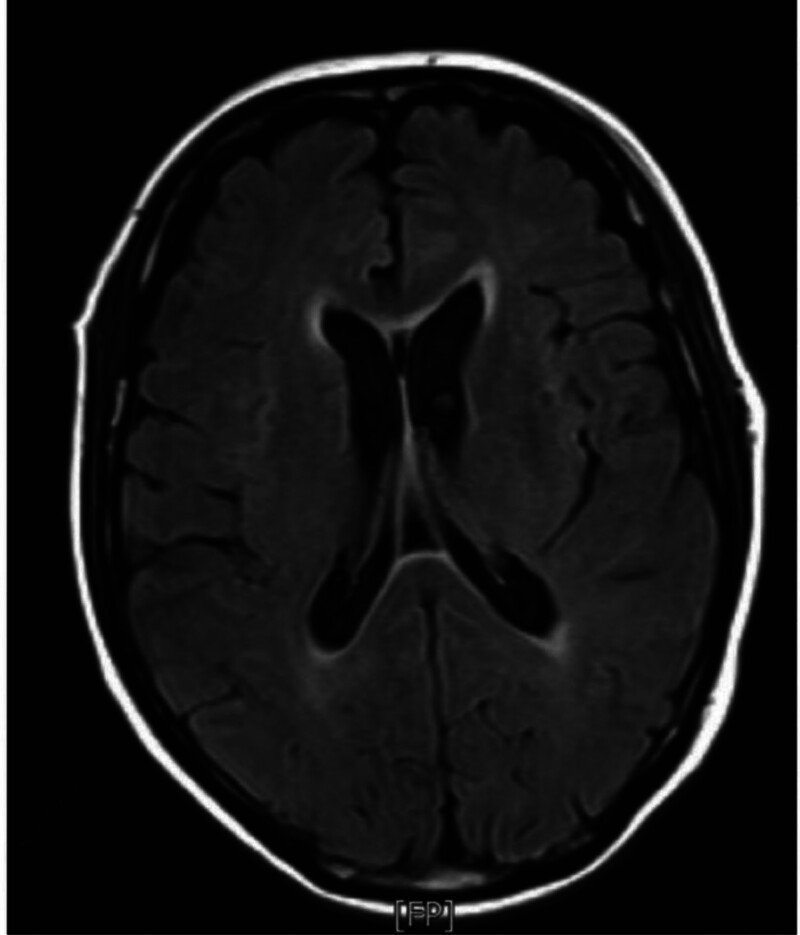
Magnetic resonance imaging (MRI) T1 weighted sequences of the brain (June 6, 2024). MRI reveals abnormal hyperintense in diploe of frontal, parietal, temporal bone, and occipital bones, leukodystrophy signal in both sides of the lateral ventricles.
